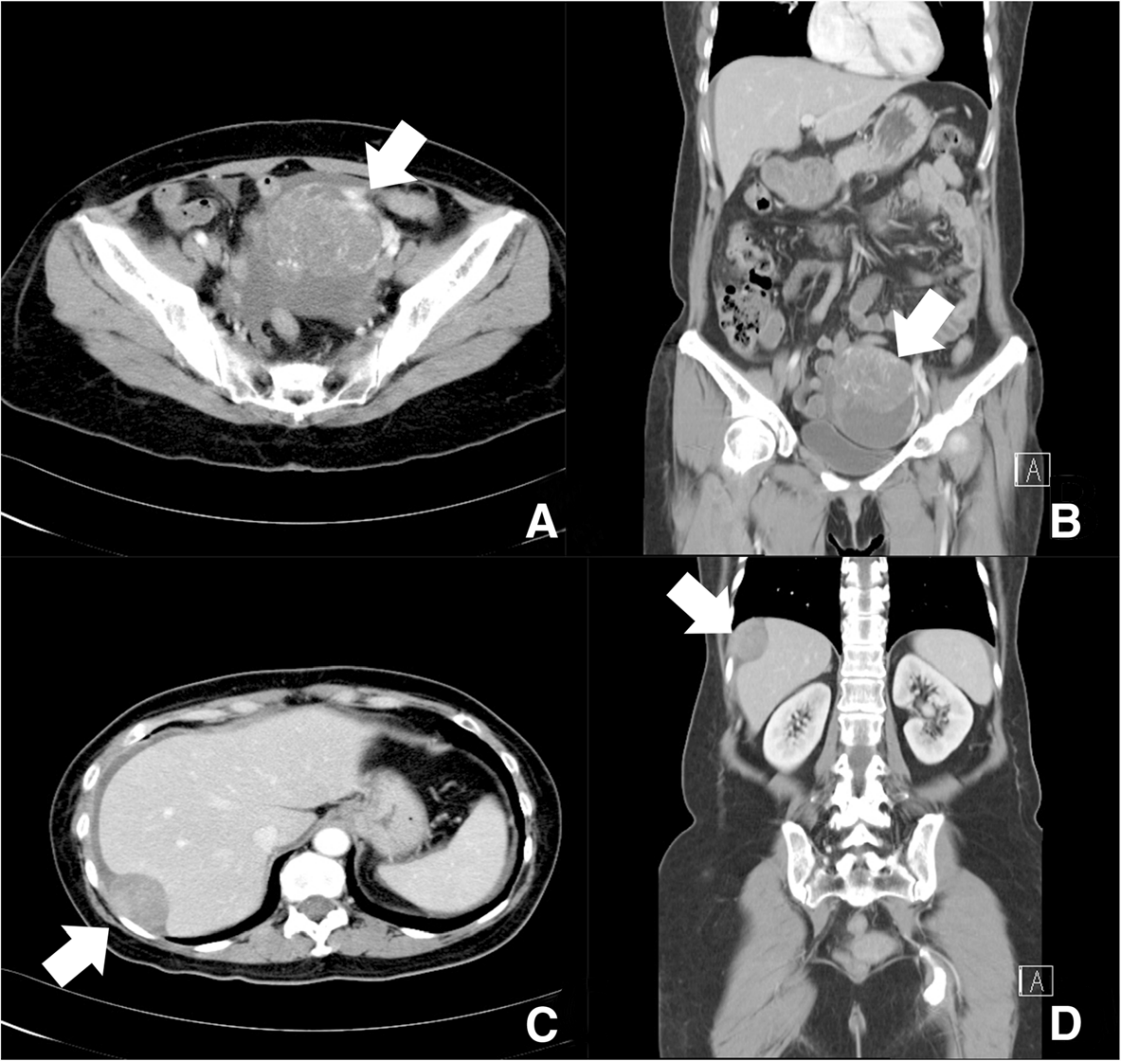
Computed tomography (a) axial and (b) coronal view showing a heterogenous mass lesion measuring 9 cm in diameter that probably developed from the left adnexa. c axial and (d) coronal view showing a well-defined mass measuring 4 cm in diameter located at the right subphrenic region
